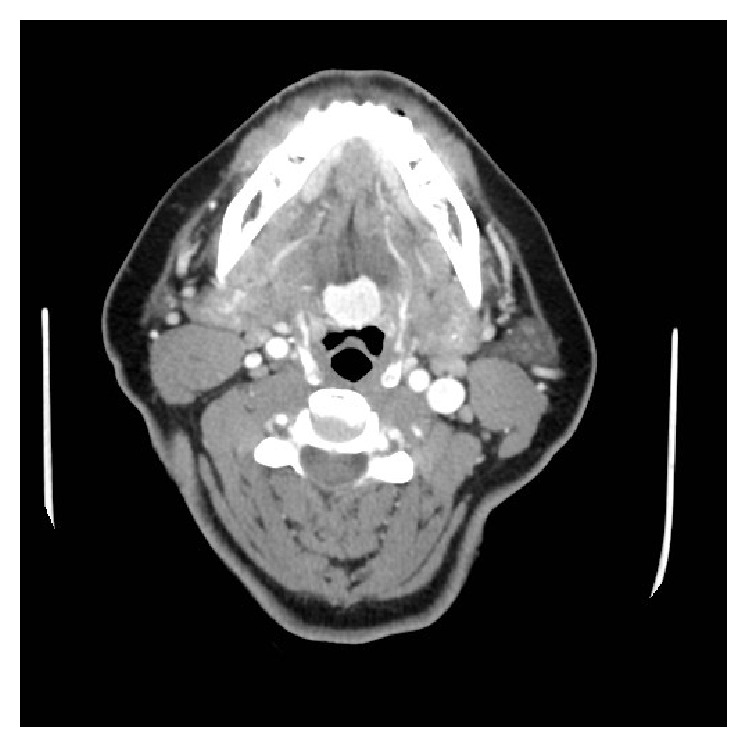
Axial CT neck showing a lingual thyroid.
